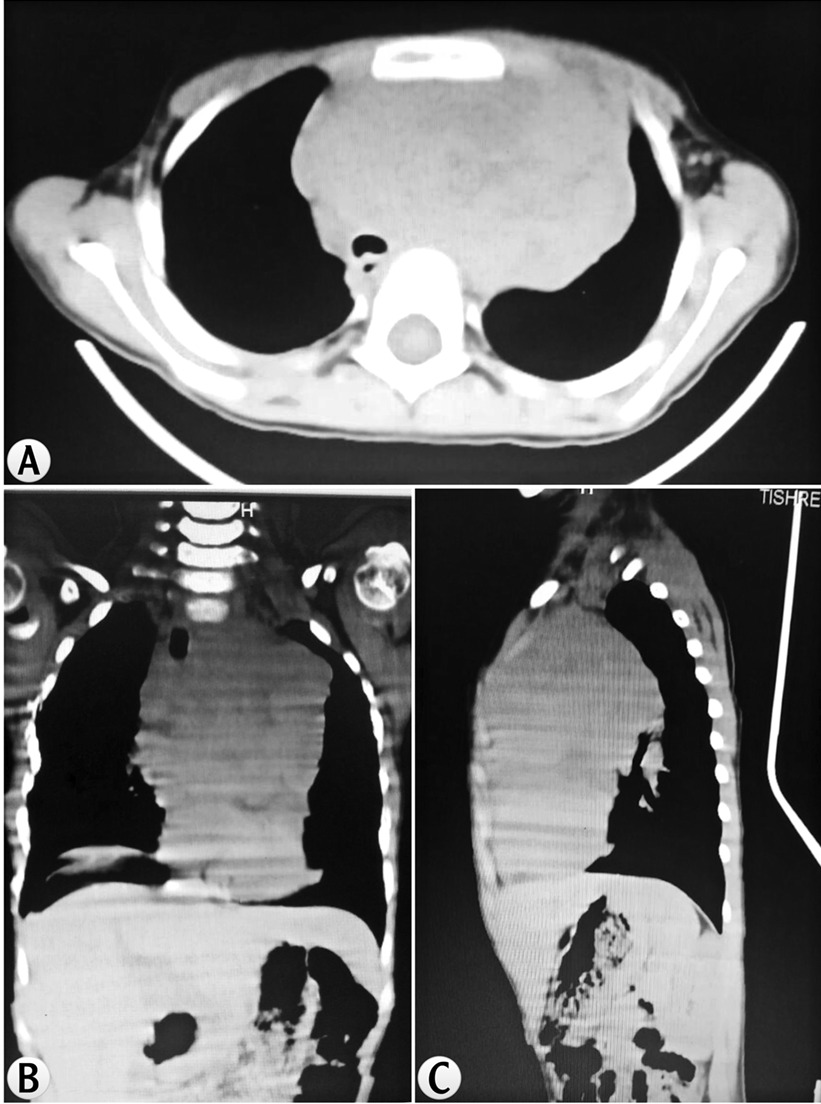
CT findings in case 1. The axial (a), coronal (b), and sagittal (c) CT planes show mediastinal expansion due to the big tumor mass, which is going up and out through the thoracic outlet, pushing the trachea to the right, narrowing the left bronchus
